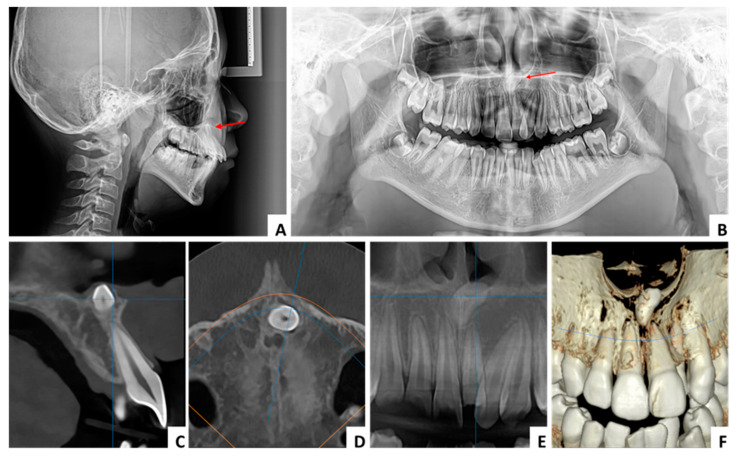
Radiological diagnostic methods—conventional 2D radiographs vs. CBCT in a case of included mesiodens: (A) lateral cephalometric—mesiodens highlighted by the red arrow, (B) OPGs—esiodens highlighted by the red arrow, (C) CBCT cross-sectional view, (D) CBCT axial view, (E) CBCT panorama view, (F) CBCT 3D reconstruction.
